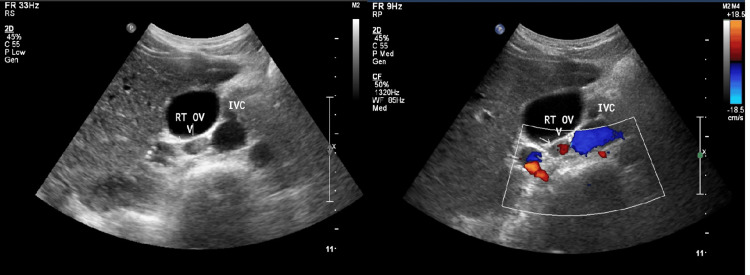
B mode and color Doppler ultrasound images The images demonstrate an echogenic thrombus within the right ovarian vein, reaching but not extending inside the inferior vena cava (IVC).
